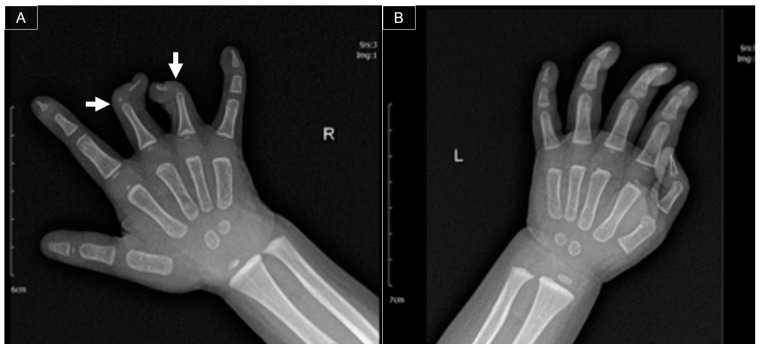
Bilateral hand X-rays ((A) right hand; (B) left hand) indicating hypoplasia in the middle phalanges and angulation deformities (arrows) in the distal phalanges of the right third and fourth fingers.
